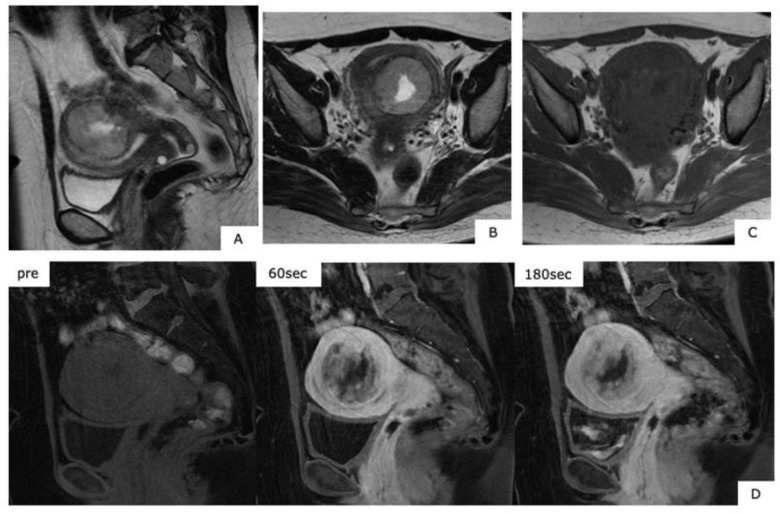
Magnetic resonance images of low-grade endometrial stromal sarcoma (age, 41 years). (A): T2-weighted sagittal section, (B): T1-weighted axial section, (C): T1-weighted axial section, (D): dynamic MRI contrast sagittal section. Within the uterine posterior wall myometrium, in T2-weighted images, there are mild high signals, with slow deep dyeing in the dynamic contrast-enhanced magnetic resonance imaging, with a finding of mass accompanied with internal necrosis. The T1-weighted images of C present faint high signals.
